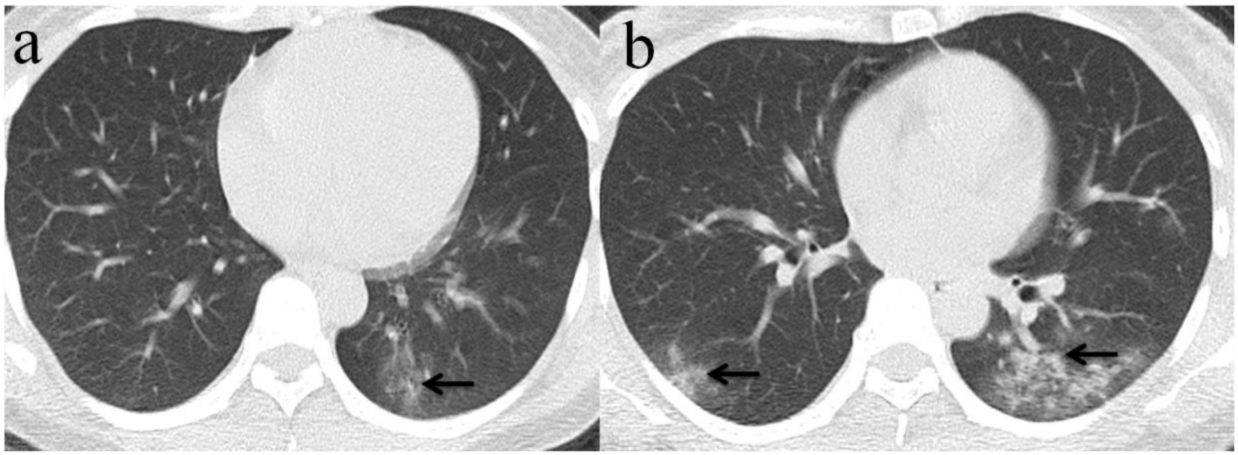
Chest CT imaging findings of COVID-19 pneumonia in a 30-year-old female patient presenting with fever (range:38.1-39℃) for four days. (a) Subpleural GGO was presented in the left lower lobe with initial negative RT-PCR (arrow);(b) 5 days later, there was an enlarged region of GGO and consolidation(arrow) presented in lower lobes of bilateral lung while RT-PCR test turned positive. (arrow).
